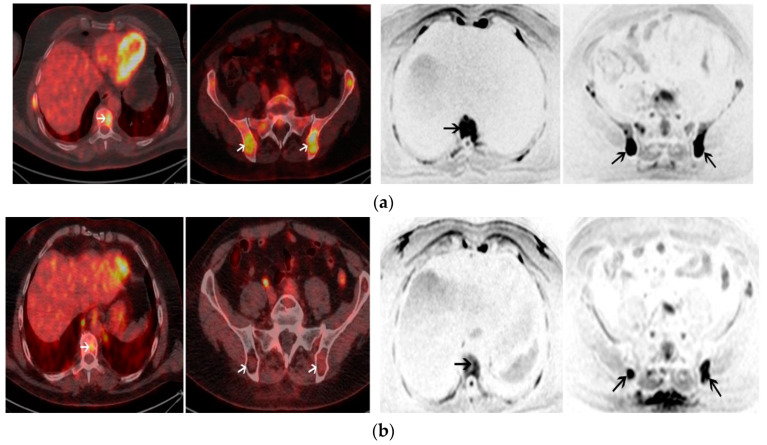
Positron emission tomography-computed tomography (PET-CT) and whole-body diffusion-weighted imaging (WB-DWI) of a 55-year-old male with IgA kappa multiple myeloma. (a) Axial images of baseline PET-CT (left panel) show multiple focal lesions with high uptake including one lesion involving T9 and two lesions involving the ilium (white arrows). Axial images of baseline WB-DWI (right panel) show lesions with restricted diffusion (b = 800 s/mm^2^) also involving T9 and the ilium with an apparent diffusion coefficient ADC of 920 μm^2^/s (black arrows). (b) Positive post-autologous stem cell transplantation (ASCT) PET-CT (left panel) shows a persistent uptake of T9 (maximum standardized uptake value > liver uptake) and the regression of uptakes within lesions involving the ilium. Positive post-ASCT WB-DWI (right panel) shows a regression of the restricted diffusion in T9 but the persistence of two lesions in the ilium with restricted diffusion and ≤25% increase in the ADC values (ADC = 1000 μm^2^/s). The subject was in complete remission after ASCT but relapsed 16 months after ASCT. Reprinted adapting the caption from Mesguich et al. [45] under the terms and conditions of the Creative Commons Attribution 4.0 International License.
